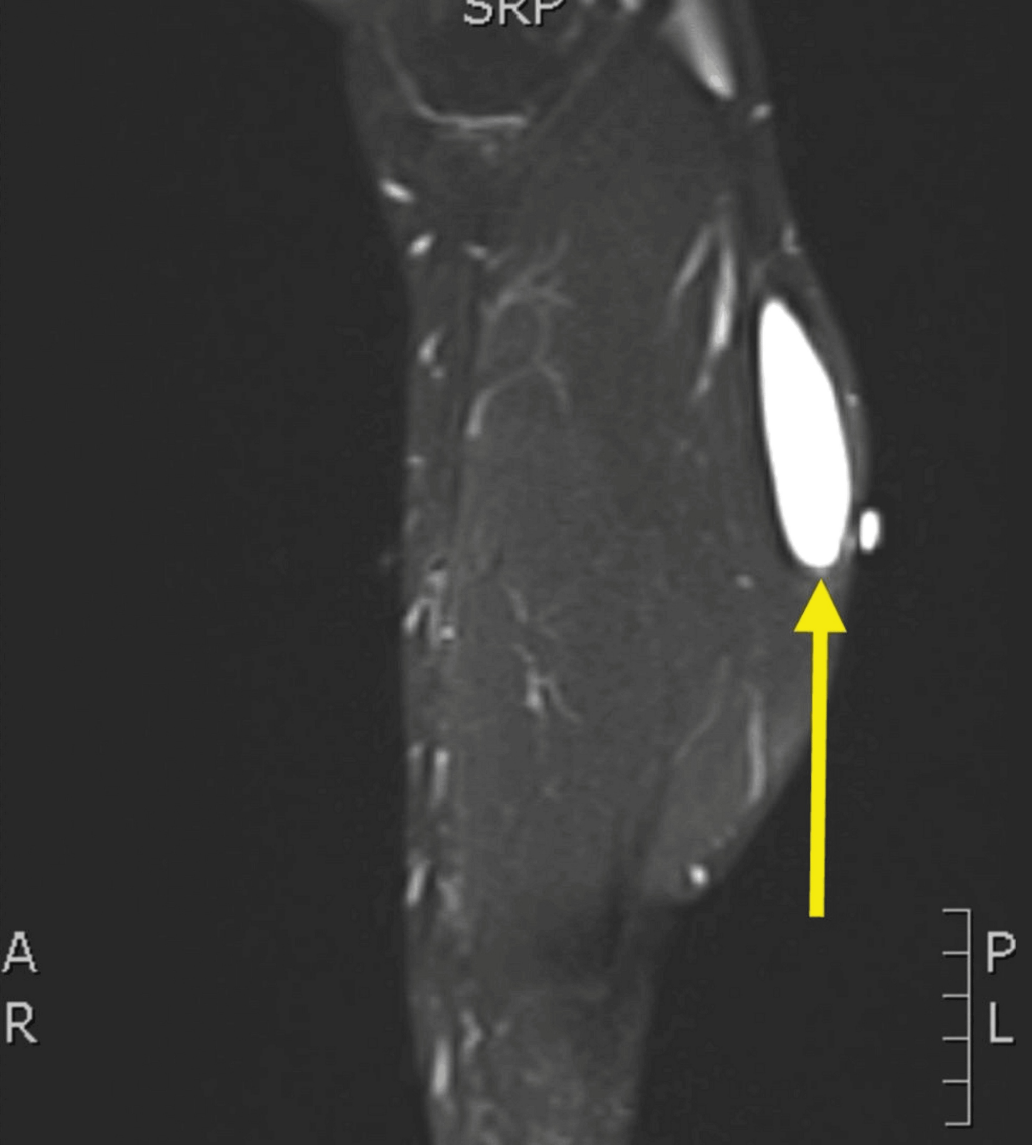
Non-contrast MRI sagittal (SAG) T2 fat suppressed (FS) image of the patient’s enhancing fluid collection demonstrating distal positioning and extramuscular extension.
